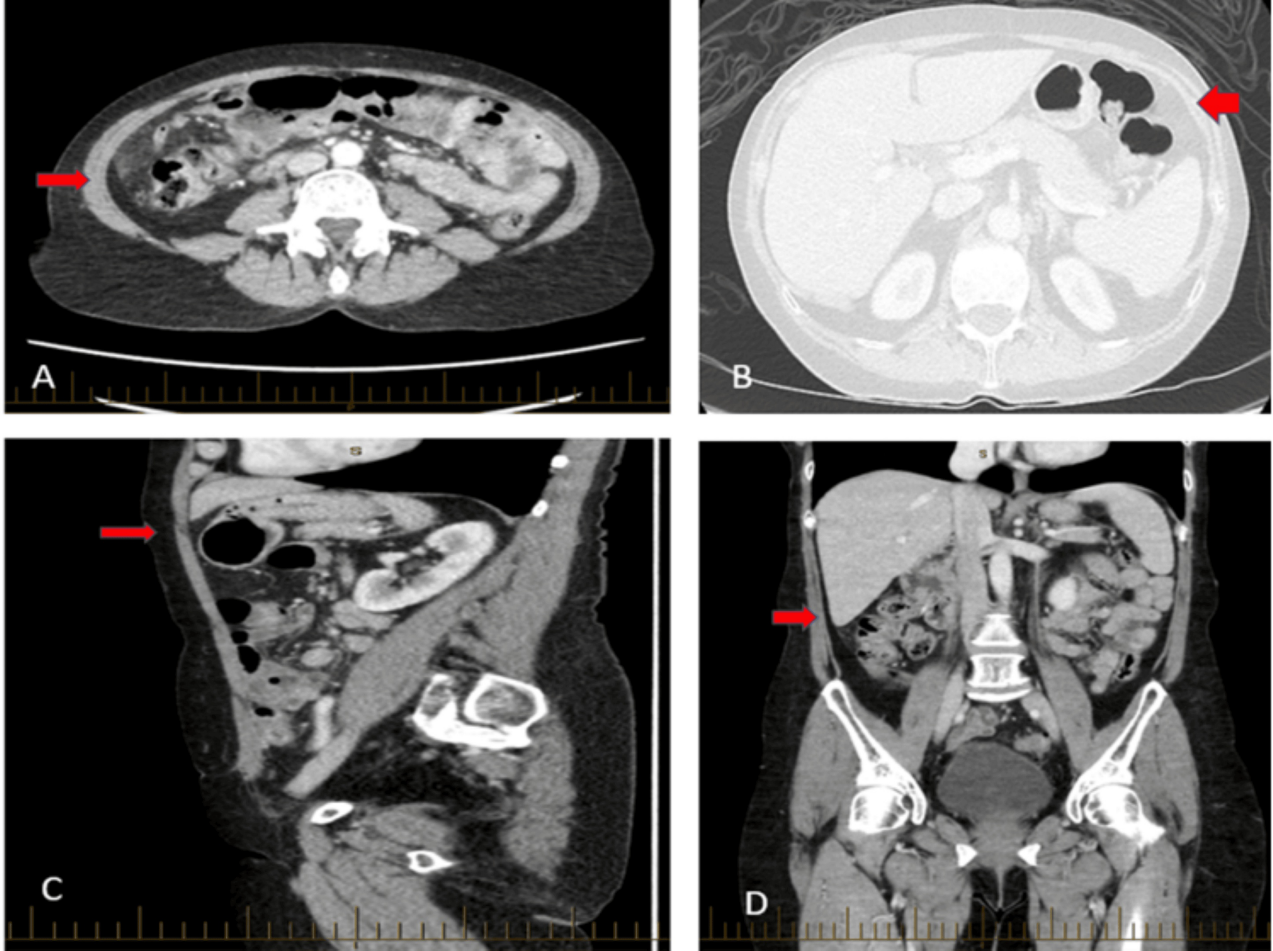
Computed tomography showing the caecum is displaced into the right upper abdomen, without distension of the proximal ascending colon
